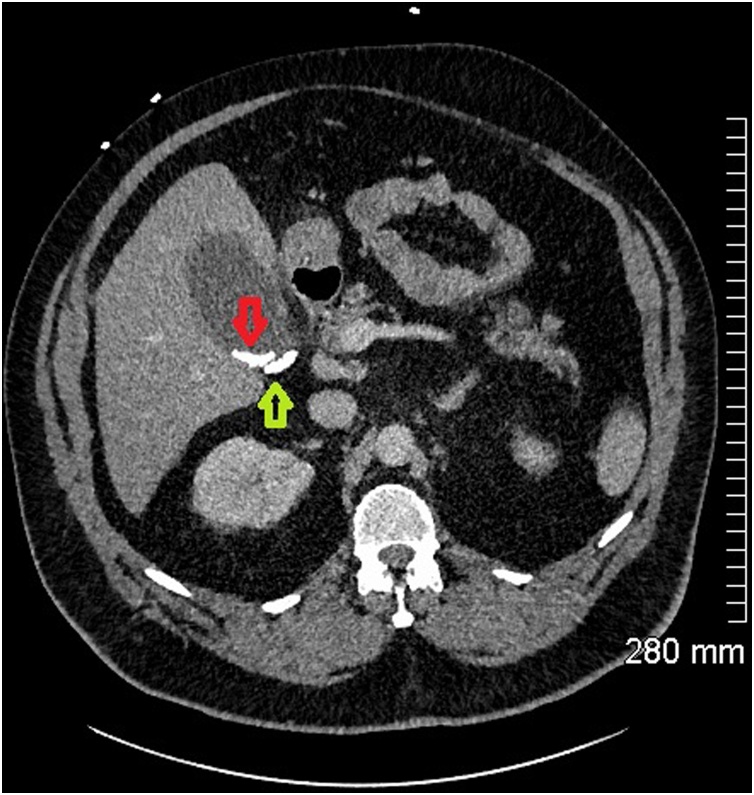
Axial CT abdomen showing calcified gallstones in the gallbladder (red arrow) and cystic duct (green arrow).
